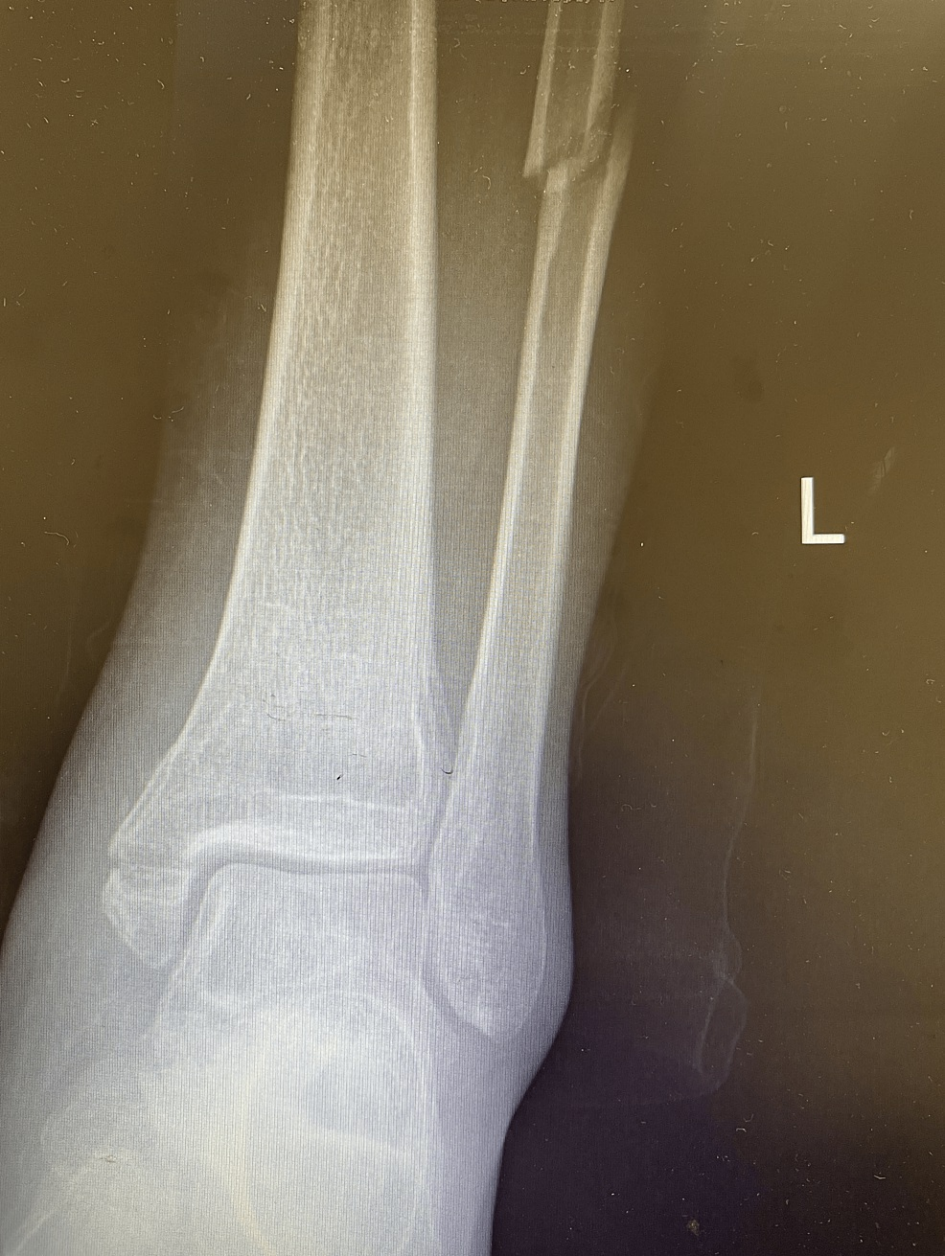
Anteroposterior ankle radiograph of a 68-year-old male presenting an ankle PER IV fracture.
